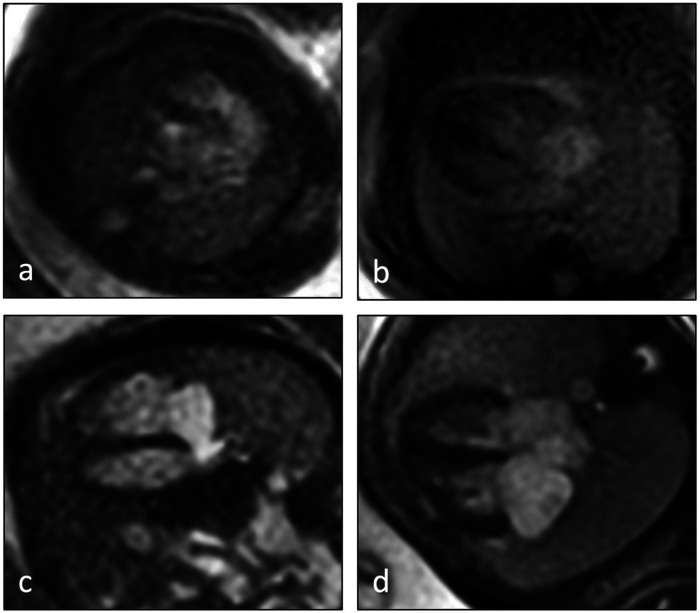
Examples of image quality scores of fetal cardiovascular MRI 4-chamber views according to the applied 4-point scale: (a) image score 1 = low quality (anatomical structures not delineable with certainty, strong artifacts), (b) image score 2 = moderate quality (anatomical structures recognizable with artifacts), (c) image score 3 = high quality (reliable definition of cardiac structures, minor artifacts), (d) image score 4 = excellent quality (reliable definition of cardiac structures without artifacts).
